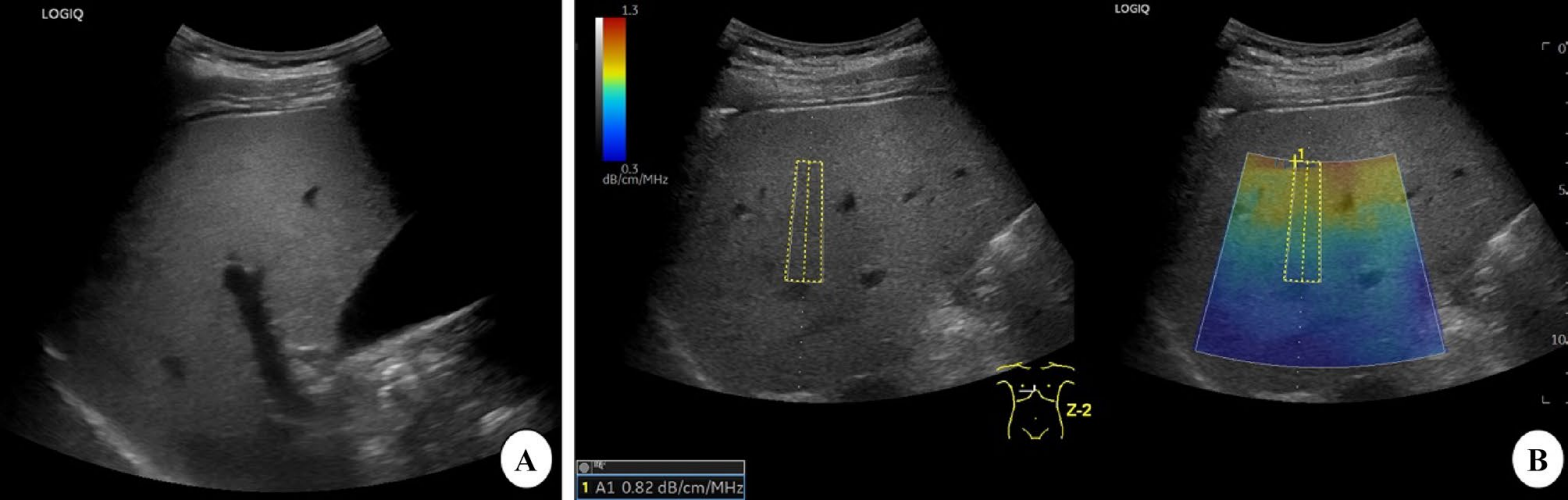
B-mode ultrasound (US) and ultrasound-guided attenuation parameter (UGAP) measurement in a 32-year-old man with hepatic steatosis. A. B-mode US imaging shows increased hepatic echogenicity with impaired visualization of periportal echogenicity and visible diaphragm echogenicity. Both reviewers 1 and 2 assessed the degree of steatosis as moderate. B. UGAP measurement was performed in the right lobe of the liver through an intercostal scan. The level of attenuation was color-coded and displayed in the region of interest (ROI), excluding the vascular structures. The ultrasound system automatically displays the attenuation coefficient (dB/cm/MHz).
